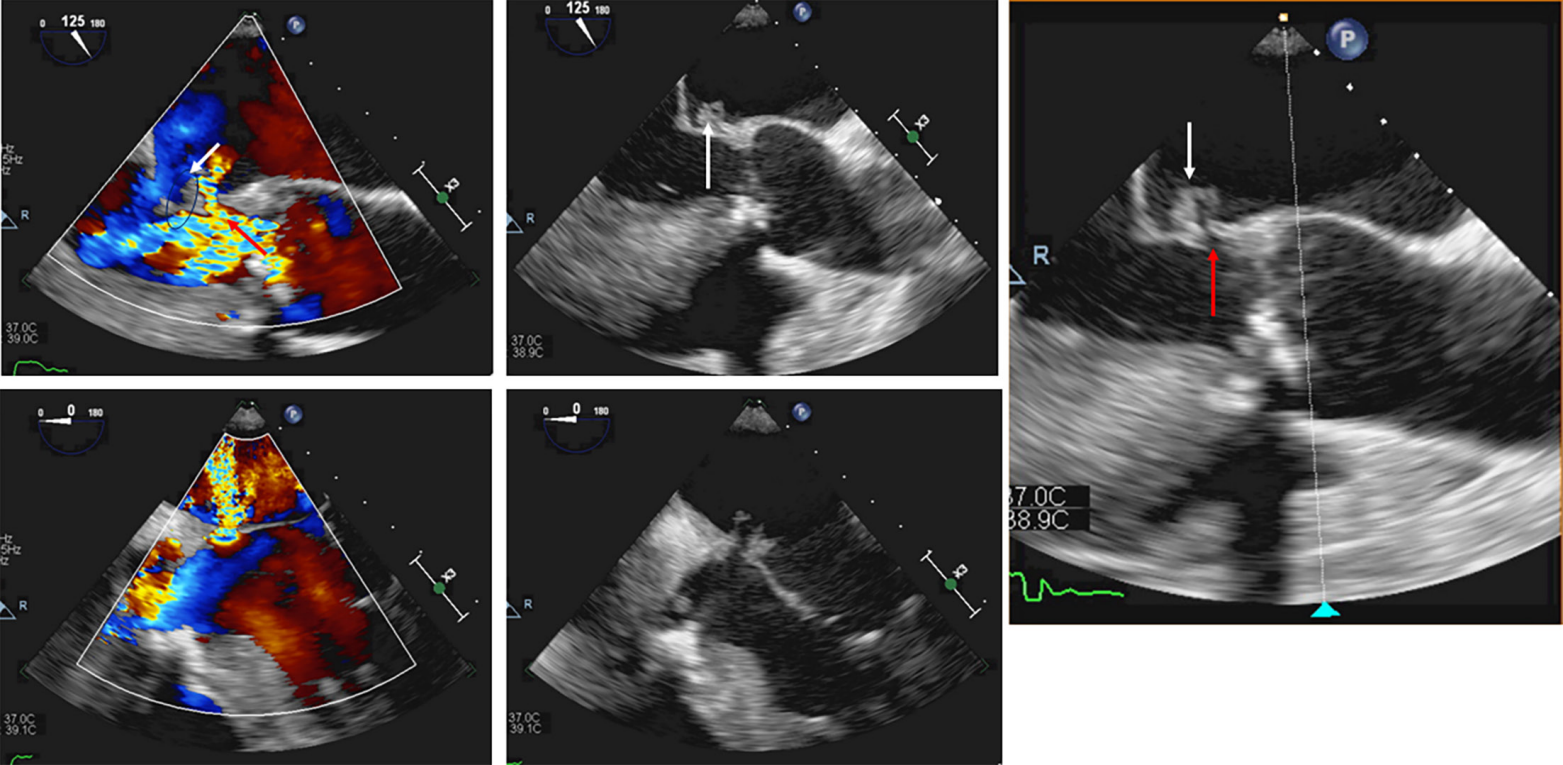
Images of the TEE examination performed in the patient showing vegetation (white arrows) and perforation (red arrows) of the mitral valve (a) and (d) are colour Doppler echocardiographic images clearly demonstrating the jet lesion through the perforation.
